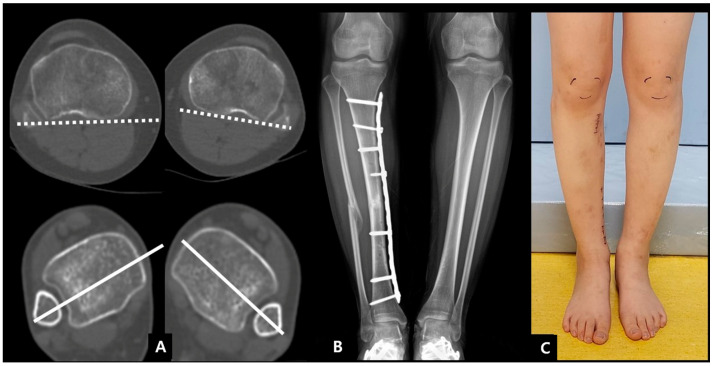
(A) Postoperative CT scan showing similar angles of rotation; (B,C) complete healing with the similar alignment of lower leg was observed 1 year postoperatively.
